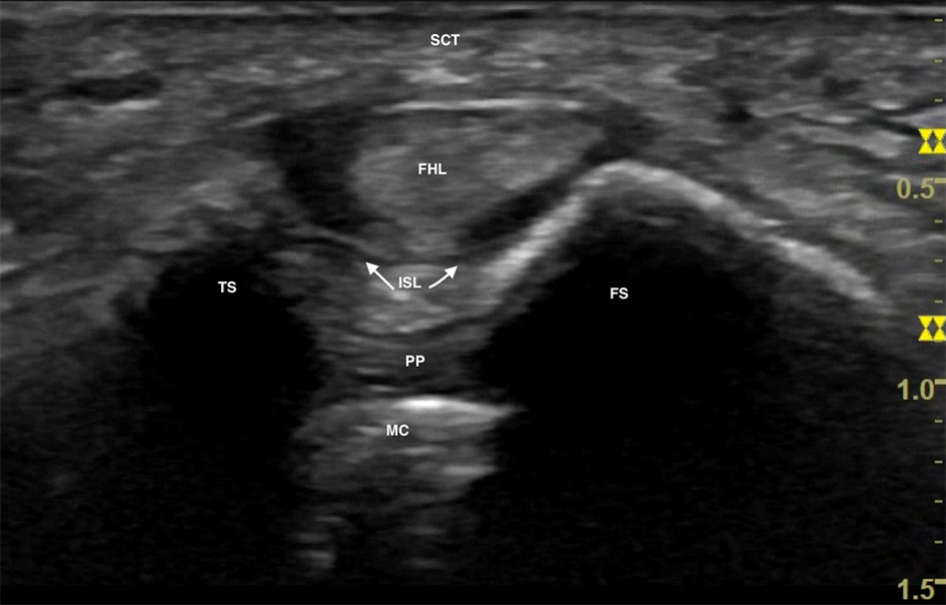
Sonographic view of the normal position of both sesamoids bones and the flexor hallucis longus (FHL) between tibial sesamoid (TS) and fibular sesamoid (FS). Note the metatarsal crest (MC) of the first metatarsal bone in line with the FHL. Intersesamoid ligament (ISL); plantar plate (PP)
